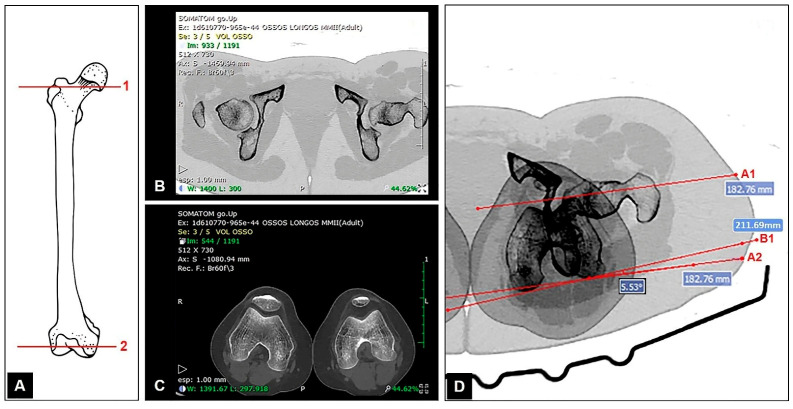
Analysis of the femoral neck anteversion angle (FNA) using computed tomography: (A) selected slices 1 and 2; (B) femoral neck slice (slice 1); (C) slice corresponding to the deepest point of the femoral trochlea (slice 2); (D) superimposition of axial images and FNA assessment (slices 1 and 2). (A1) longitudinal axis of the femoral neck; (B1) posterior transcondylar line; (A2) duplication of A1 positioned at its intersection with B1 to obtain the angle. The letters “R,” “L,” and “P” indicate laterality and anatomical position, representing right, left, and posterior, respectively.
